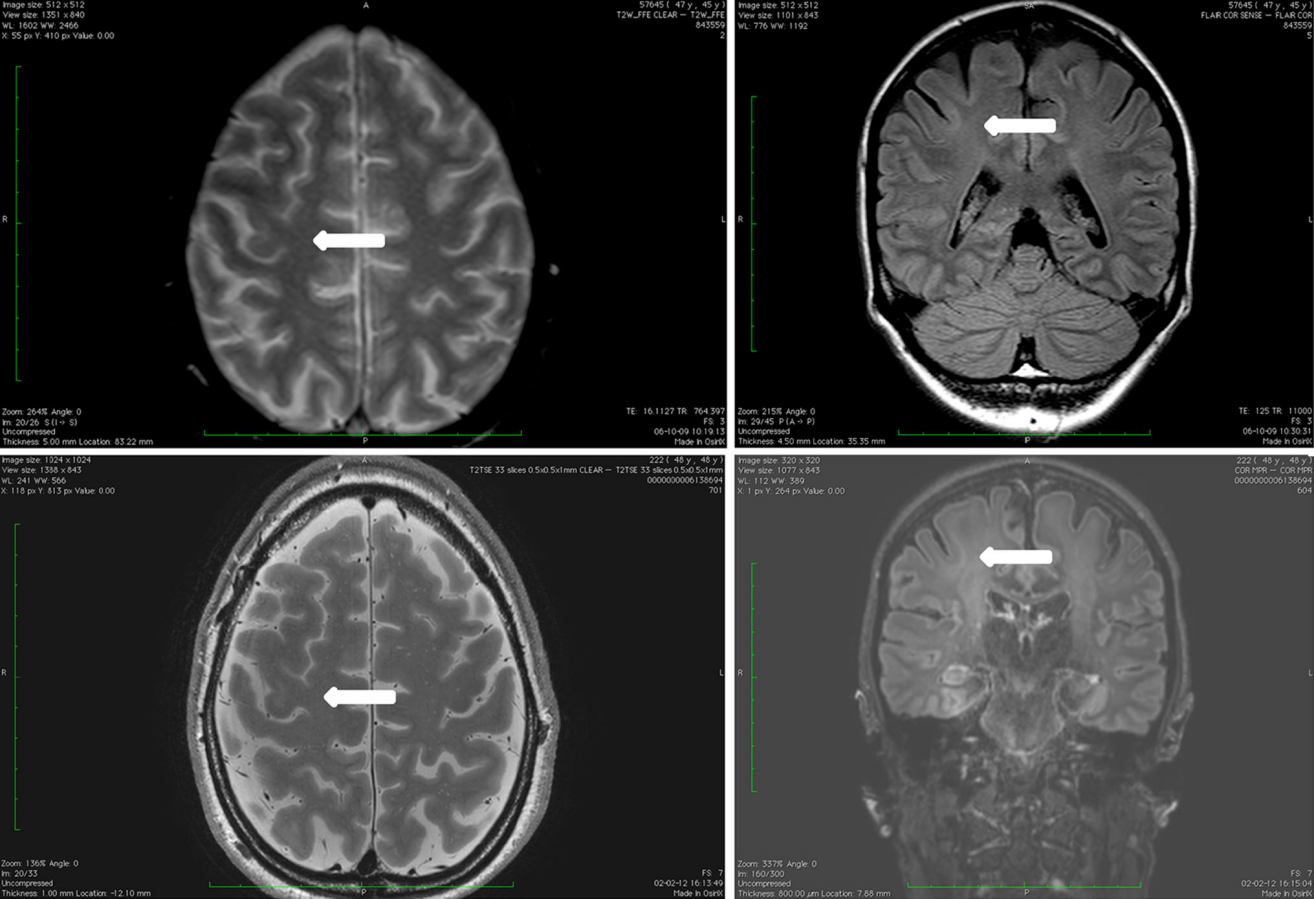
Comparison between 3 (*top*) and 7 (*bottom*) T images of a patient with known FCD *right hand* knob to deep-of-the-sulcus. From *left* to *right* T2, FLAIR
